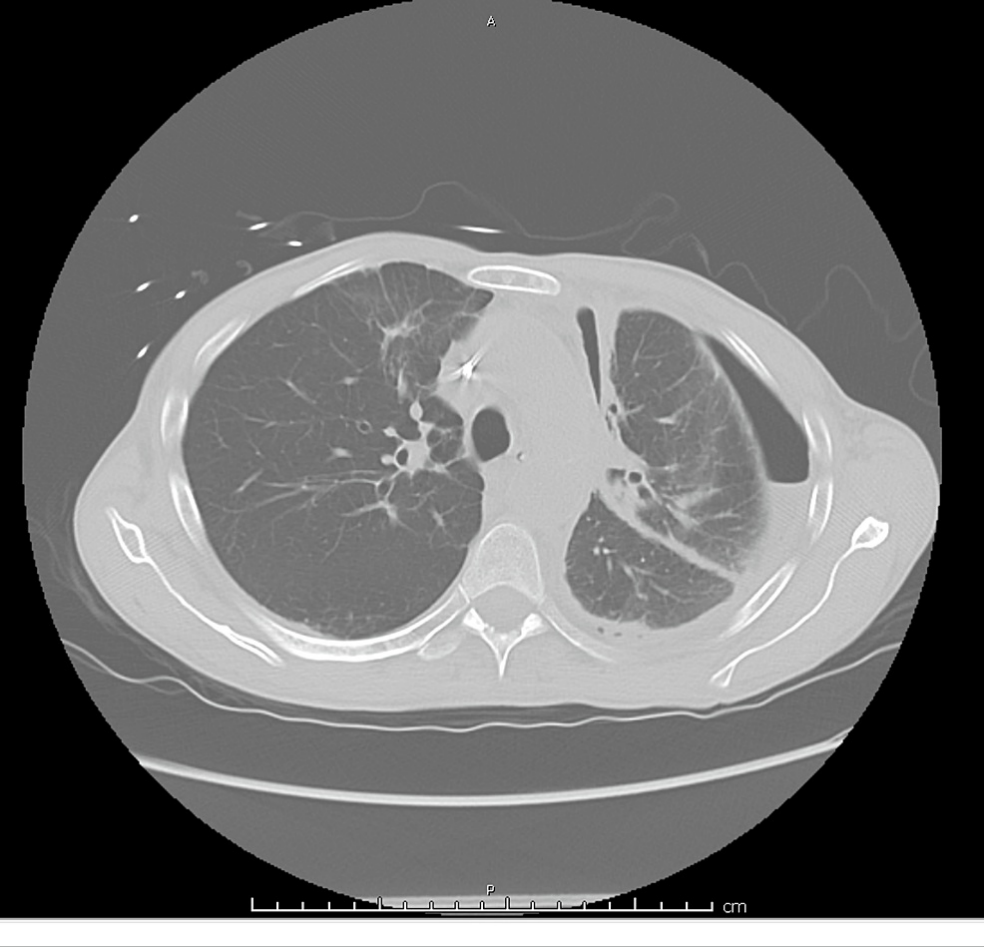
CT Chest showing entrapped lung with pneumothorax ex vacuo
